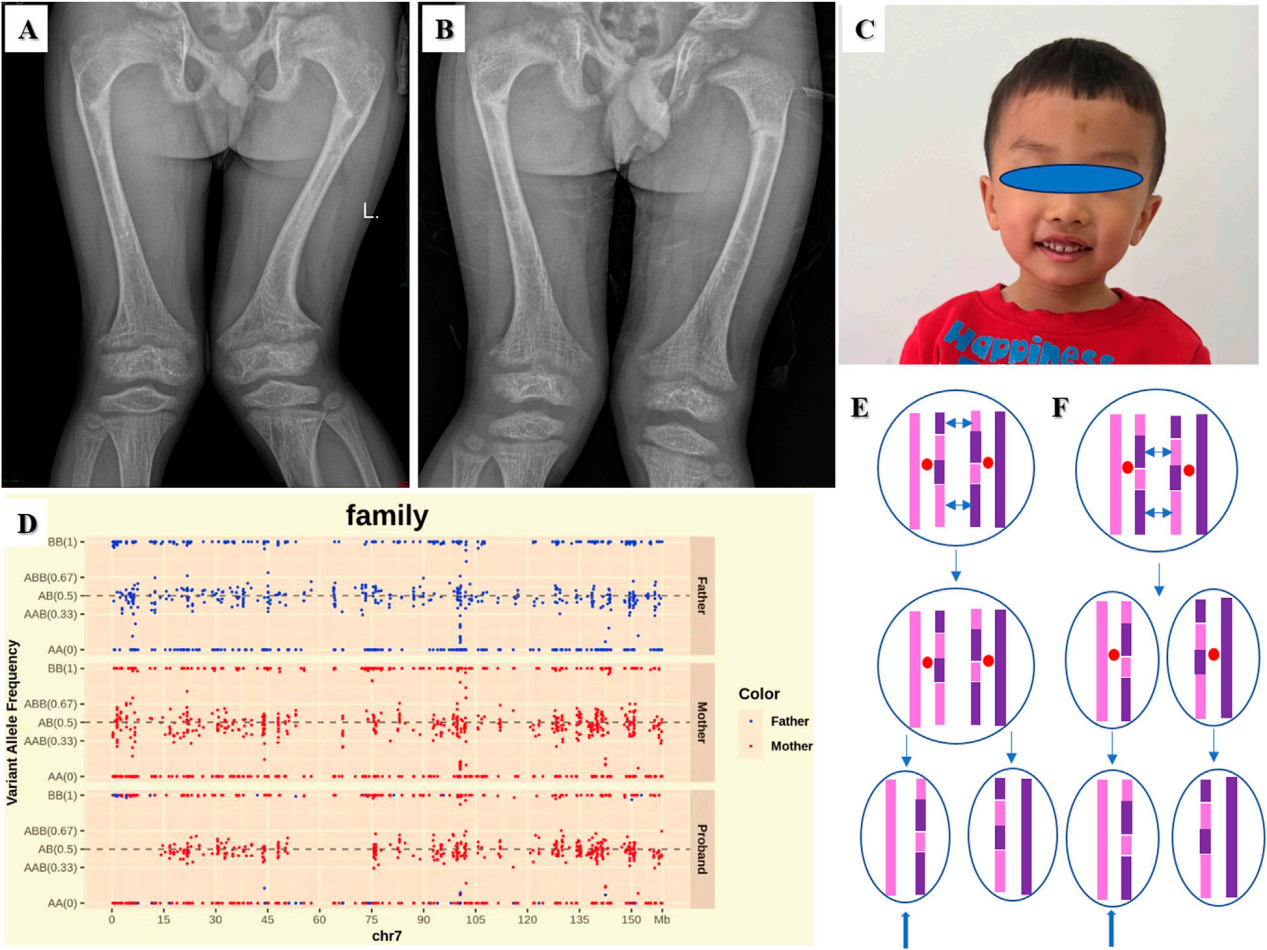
Radiographic features of the fourteenth proband (A) and his younger brother (B): frayed and cupped metaphyses, pseudofractures, and osteoporosis in the femurs. (C) The facial feature of the nineteenth proband. (D) SNPs analysis based on Trio-WES revealed a mixture of maternal isodisomy and heterodisomy of chromosome 7. The possible mechanism of the mixture pattern of uniparental disomy (UPD) is based on recombination of maternal homologs and maternal nondisjunction at Meiosis I (E) or Meiosis II (F). The double-headed arrows show crossing over of genetic material between homologous chromosomes during meiosis. The blue arrows show a mixture of maternal isodisomy and heterodisomy of chromosome 7.
